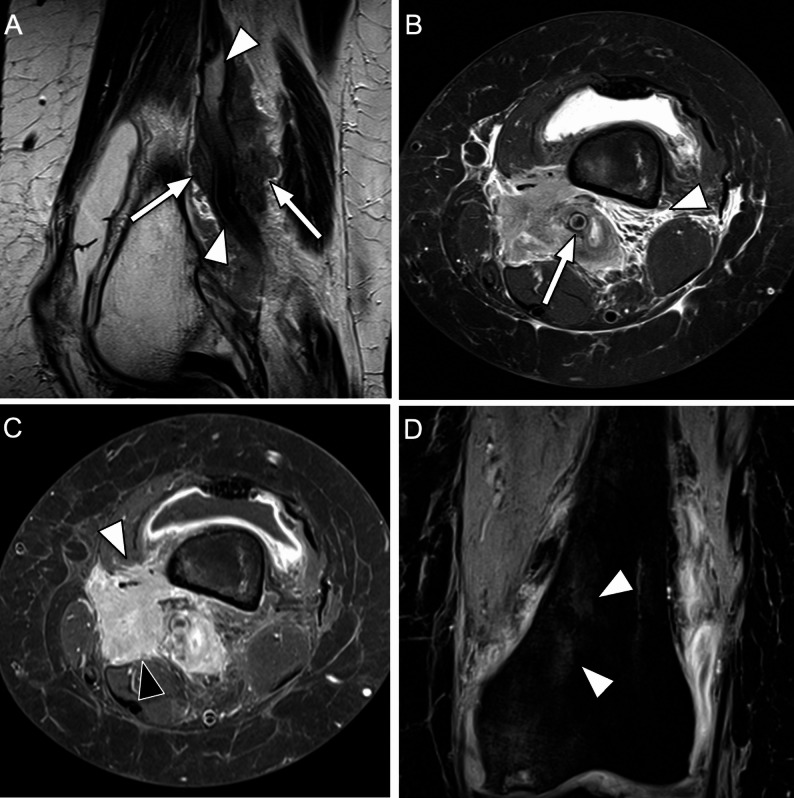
MRI of a 73-year-old female presenting with non-Hodgkin lymphoma manifestation in the popliteal fossa. A Sagittal T2-weighted image shows tumor (arrows) extending along the popliteal vessels (arrowheads). B Transversal fat-saturated PD-weighted image reveals encasement of popliteal vessels (arrow) and peritumoral edema (arrowhead). C Transversal contrast enhanced fat-saturated T1-weighted image shows infiltration of anterior (white arrowhead) and posterior (black arrowhead) femoral compartments D Coronal contrast enhanced T1-weighted image reveals patchy enhancement of medullary space (arrowheads) without cortical destruction
